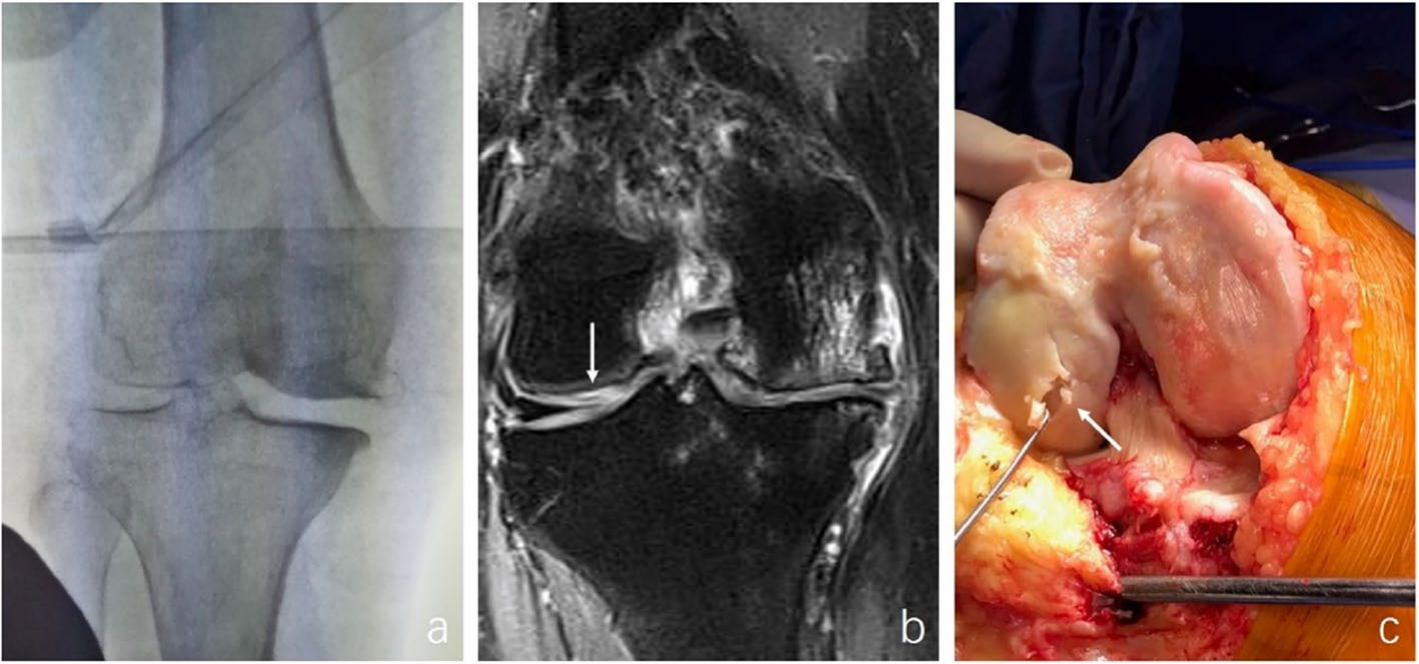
a A 69-year-old female whose valgus stress radiographic LJSW was normal, indicated intact articular cartilage in lateral compartmental. b Coronal plane on MRI showed intensive signal (arrow) in lateral femoral weight-bearing area cartilage with Recht grade 3. c The high-grade cartilage injury (arrow) was confirmed intraoperatively
